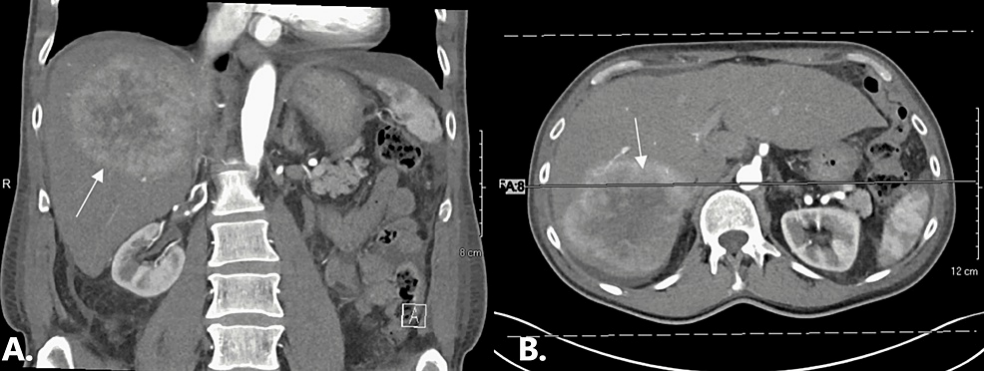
Abdomen computed tomography with liver protocol (A) Coronal view showing a 12.3 × 9.9 × 9.8 cm arterial enhancing mass with washout and pseudo-capsule centered in the posterior right hepatic lobe (arrow); (B) sagittal view showing the same enhancing mass in the posterior right hepatic lobe (arrow)
